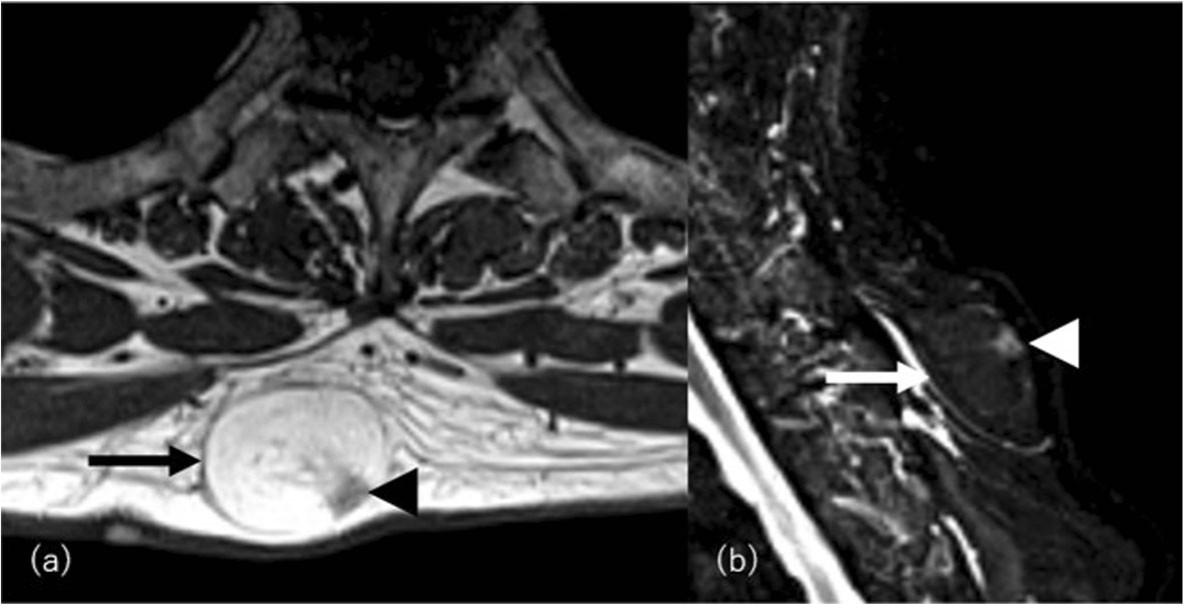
Spindle cell lipoma in a 68-year-old man with a painless mass on the posterior neck. a Axial T1-weighted image and b sagittal STIR image show a subcutaneous, encapsulated fatty mass (arrows) with amorphous non-fatty signal area (arrowheads) representing intermingled components such as collagen fibers, myxoid matrix, and vascular elements
